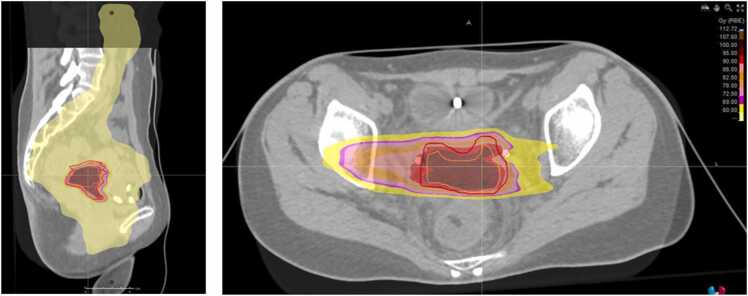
Summed dose distribution of the 2 treatment plans (dose from external beam photon radiation therapy and carbon ion radiation therapy - CIRT), expressed in EQD2. The photon beam plan was prescribed at 95% of the volume, while CIRT was at 50% of the volume. The nominal prescribed median total dose to the clinical target volume (CTV) exceeded 94.8 Gy(RBE). Due to the proximity of critical organs at risk, the dose was reduced in the cranial portion of the CTV. Note that the two planning CT scans were registered using different patient setup conditions.
